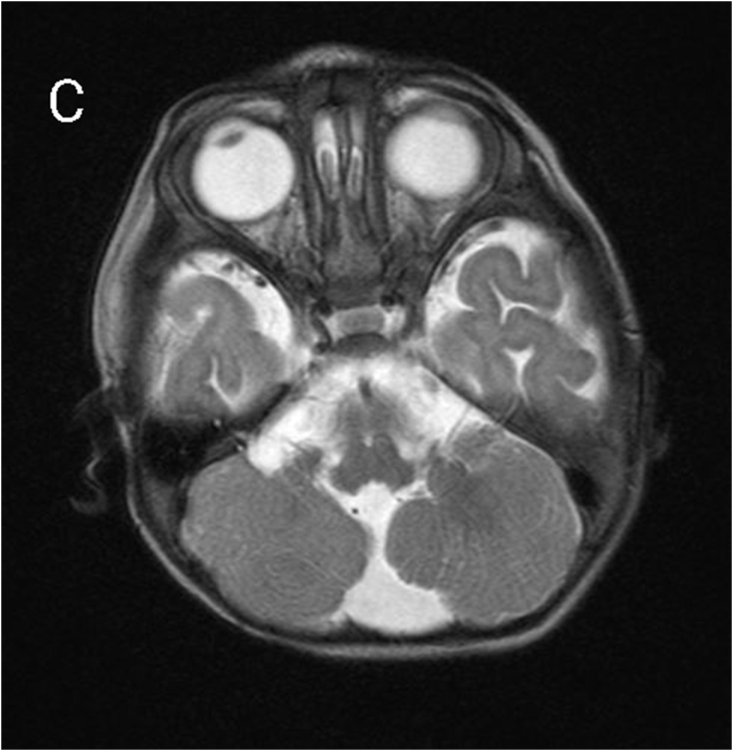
transverse T2-weighted image showing hypogenesis of the inferior vermis.
